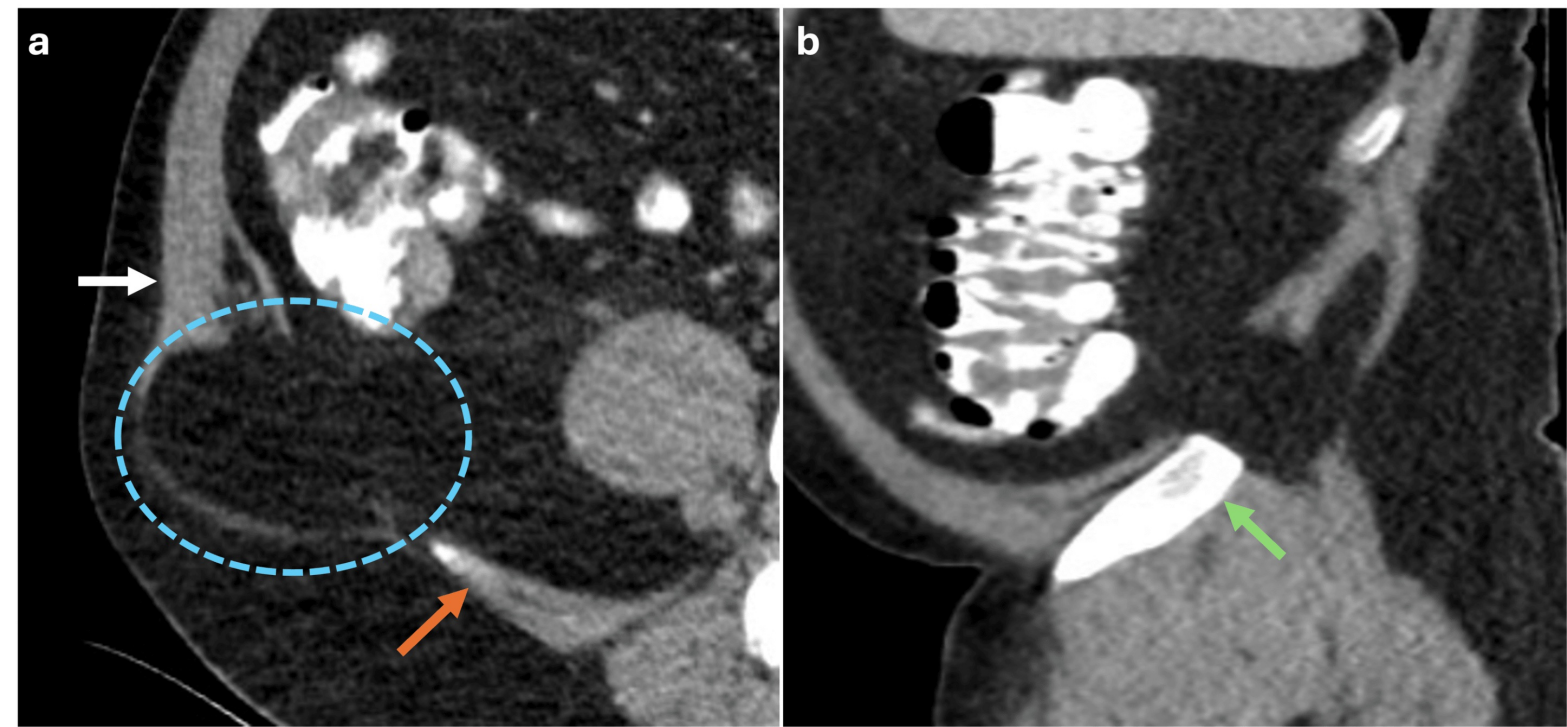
Axial and sagittal abdominal CT scan with oral contrast showing a Petit hernia (a, b) Axial and sagittal CT scan demonstrates a hernia sac with fat content (blue dotted circle) delimited laterally by the external oblique muscle (white arrow), medially by the latissimus dorsi muscle (orange arrow) and inferiorly by the iliac crest (green arrow), consistent with a Petit hernia in the lower lumbar triangle. The figure was created by the authors.
